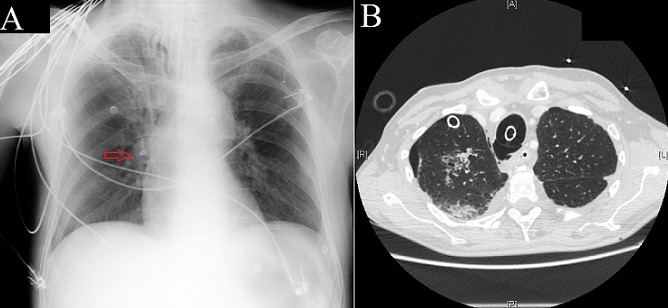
A): chest x-ray showing Sengstaken-Blakemore tube in the right bronchus just after balloon were deflated (arrow points the tip); B): chest CT scan showing right pneumothorax with chest tube, pneumomediastinum and orotracheal tube with the tracheal rupture level
